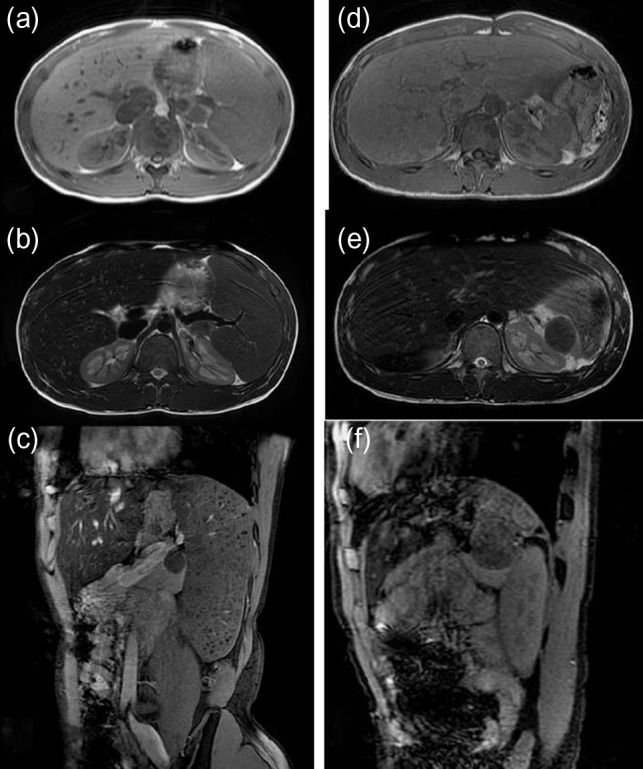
Magnetic resonance imaging (MRI) (before and after splenectomy). (a–c) Before splenectomy: MRI shows a huge spleen and a 2-cm mass in the pancreatic tail. (d–f) After splenectomy: MRI shows the mass in the pancreatic tail grows rapidly to 4 cm.
